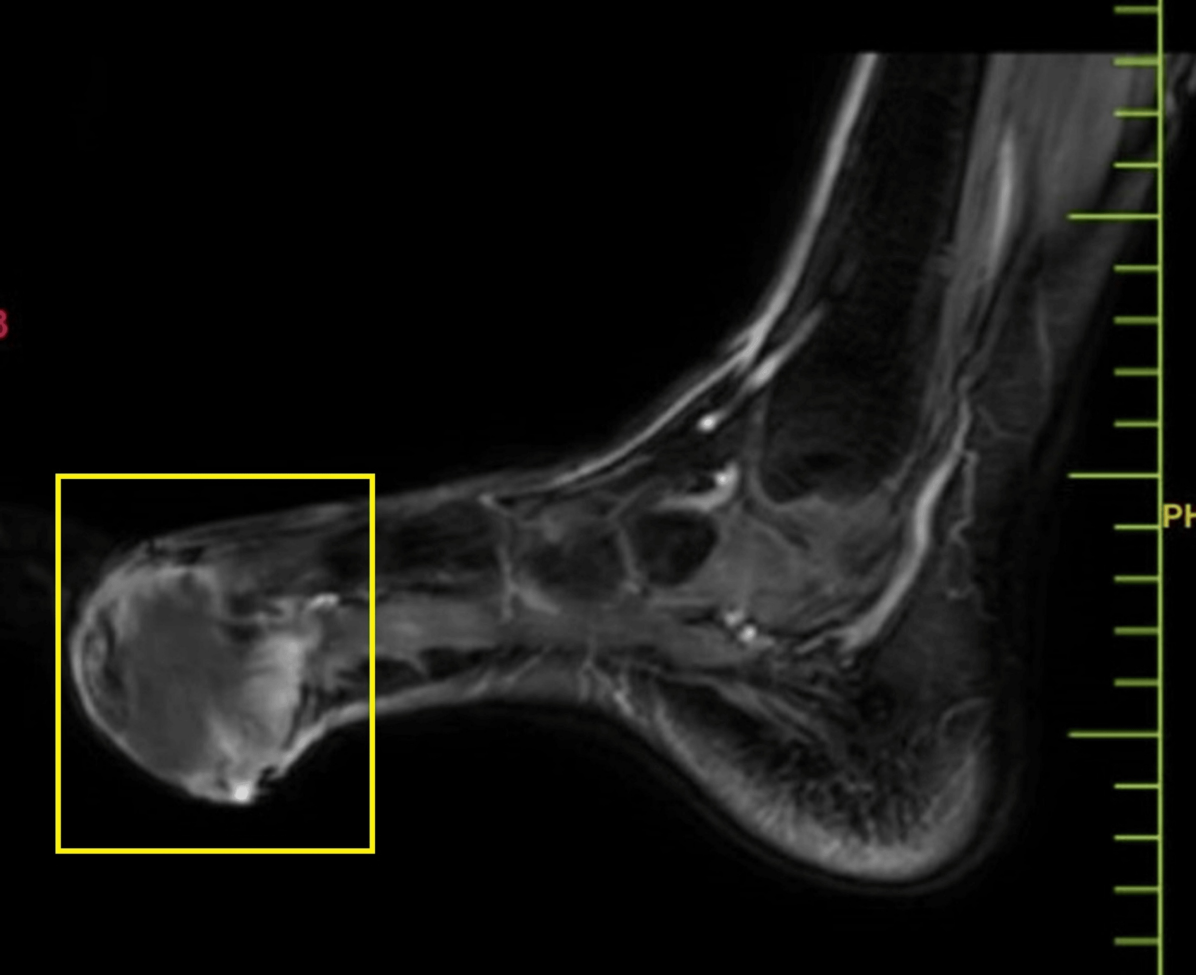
MRI of the foot (sagittal plane) MRI: magnetic resonance imaging
